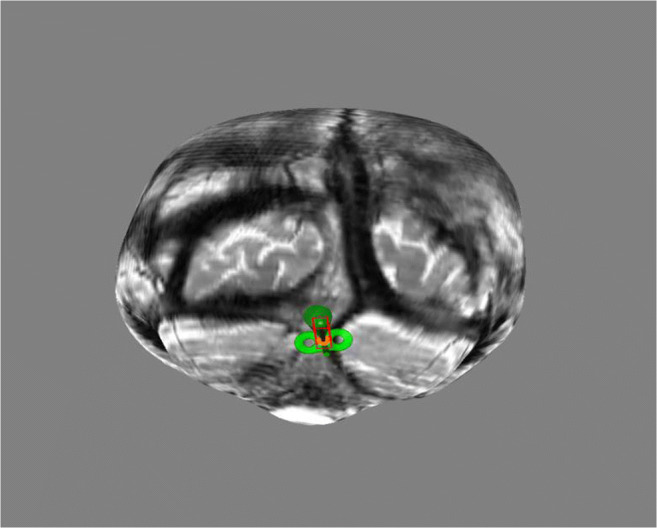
Cerebellar vermis stimulation point from a participant’s MRI using frameless functional MRI stereotaxy (Brainsight 2, Rogue Research, Montreal, Canada)
